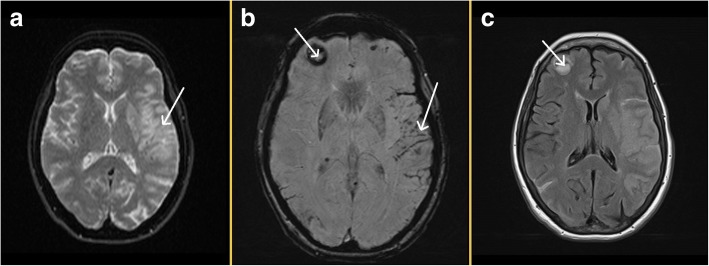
a: Established infarct in the left MCA seen in the DWI sequence, indicated by the arrow b: Right sided arrow demonstrates luxary hyperperfusion in the area of the infarct without significant hemorrhagic transformation on the SWI sequence, and left sided arrow shows a cerebral mass c: Flare sequence favours a probable cerebral metastatic deposit (arrowed)
